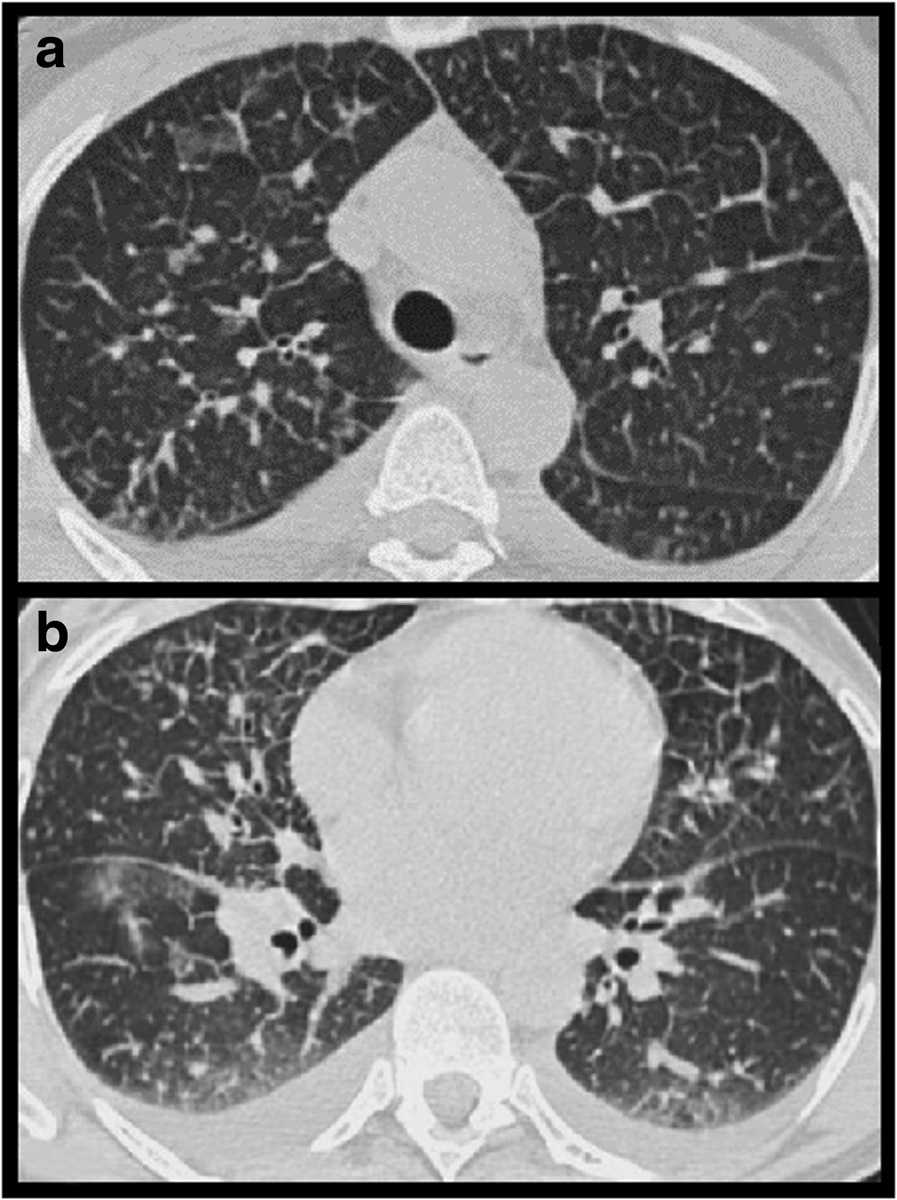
Pulmonary interstitial and alveolar oedema. Patient with adjuvant chemotherapy for breast cancer. a, b chest CT at the level of the aortic arch (a) and the right lower pulmonary vein (b) at lung windows: Diffuse bilateral reticular pattern due to interlobular septal thickening, some mild ground glass, mild thickening of bronchovascular bundles and bilateral pleura l effusions, right larger than left
